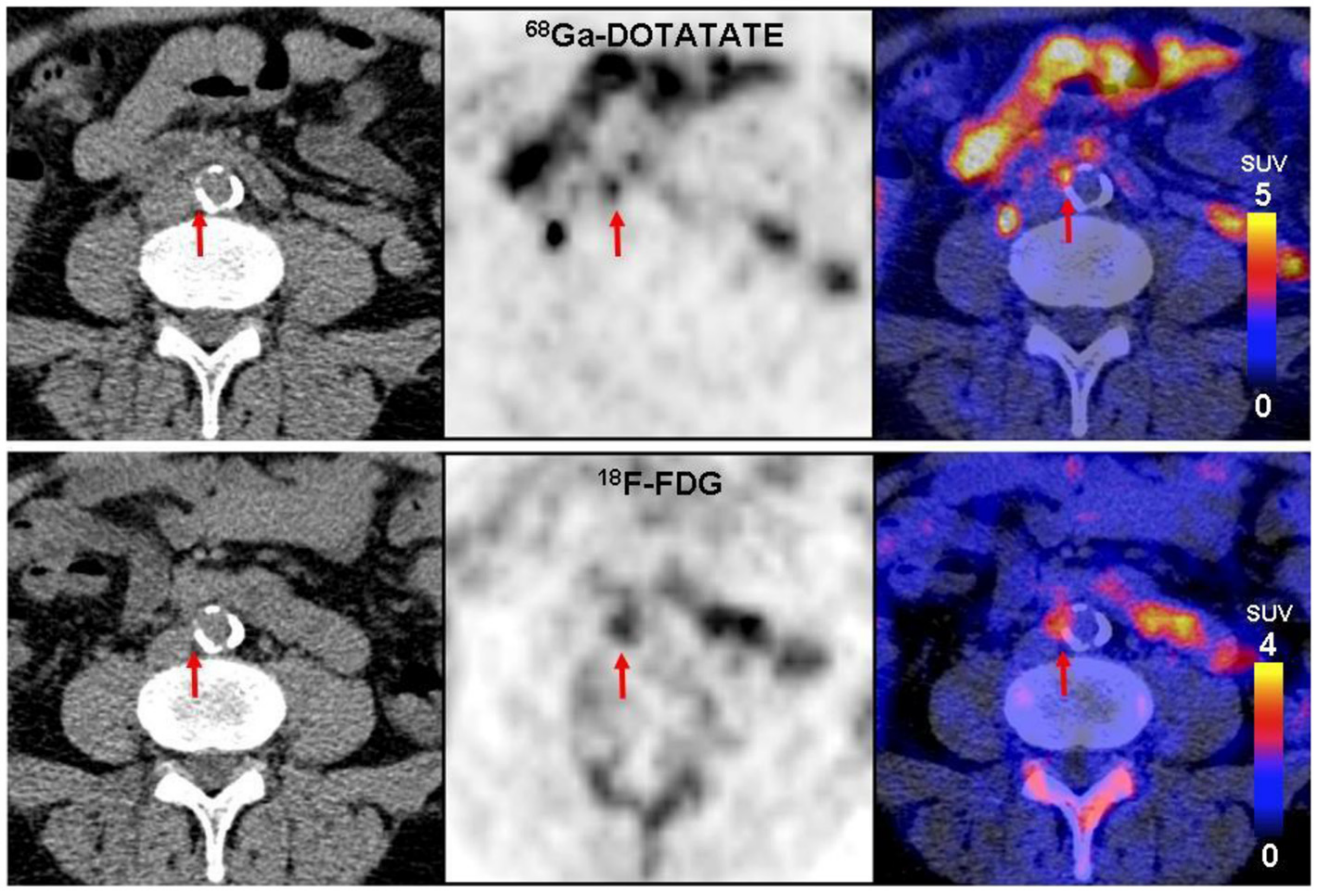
Colocalized focal vascular uptake of ^18^F-FDG and ^68^Ga-DOTATATE. Transverse views of a 61-year-old male patient with hypertension and a history of cardiovascular disease. Positive uptake is present on both ^68^Ga-DOTATATE (upper row) and ^18^F-FDG PET/CT (lower row) at the same location in the abdominal aorta of this patient (red circle). Also, serious calcification was detected at the same position. TBR_DOTATATE_ was 6.18, while TRB_FDG_ was 2.42.
